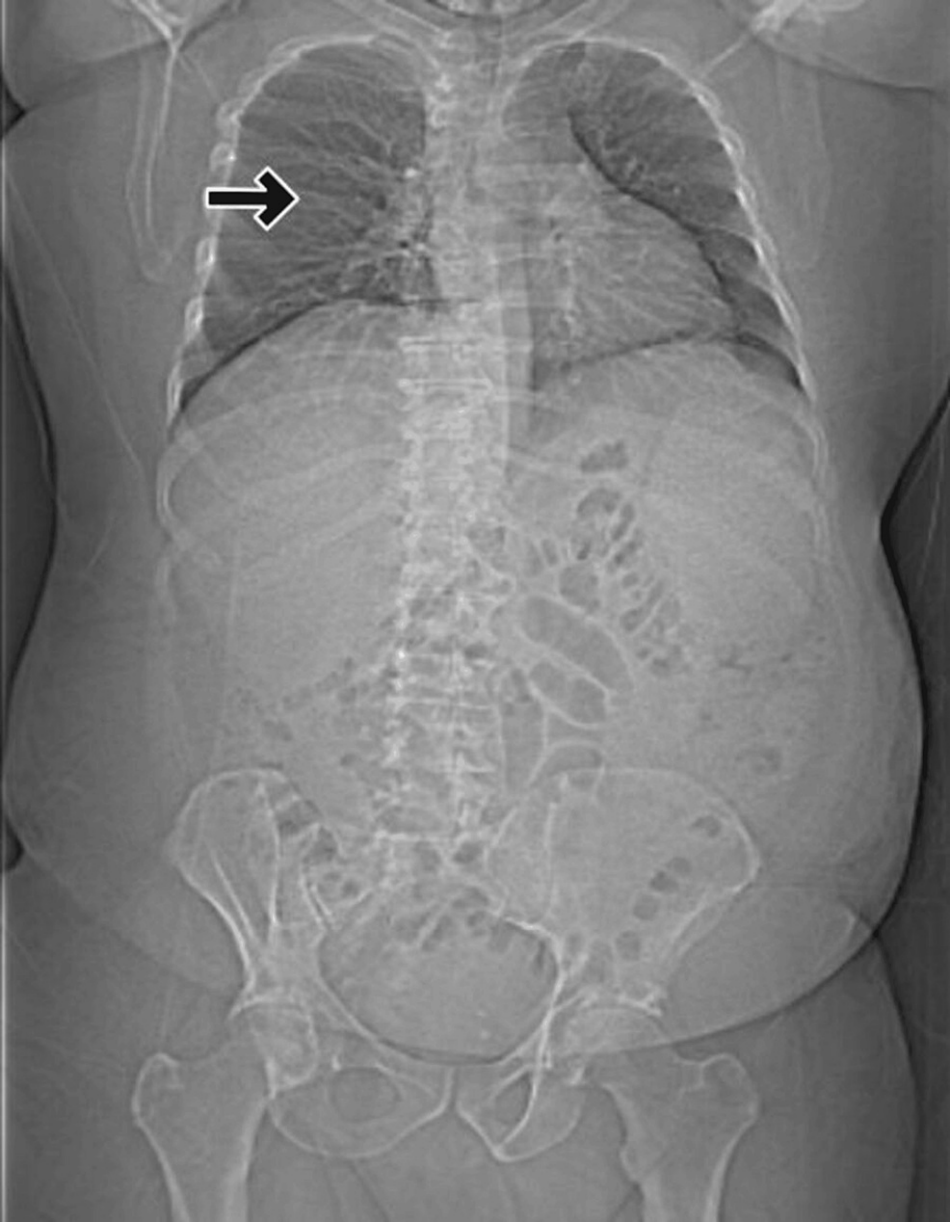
X-ray of the abdomen and pelvis with arrow demonstrating extensive lymphadenopathy
